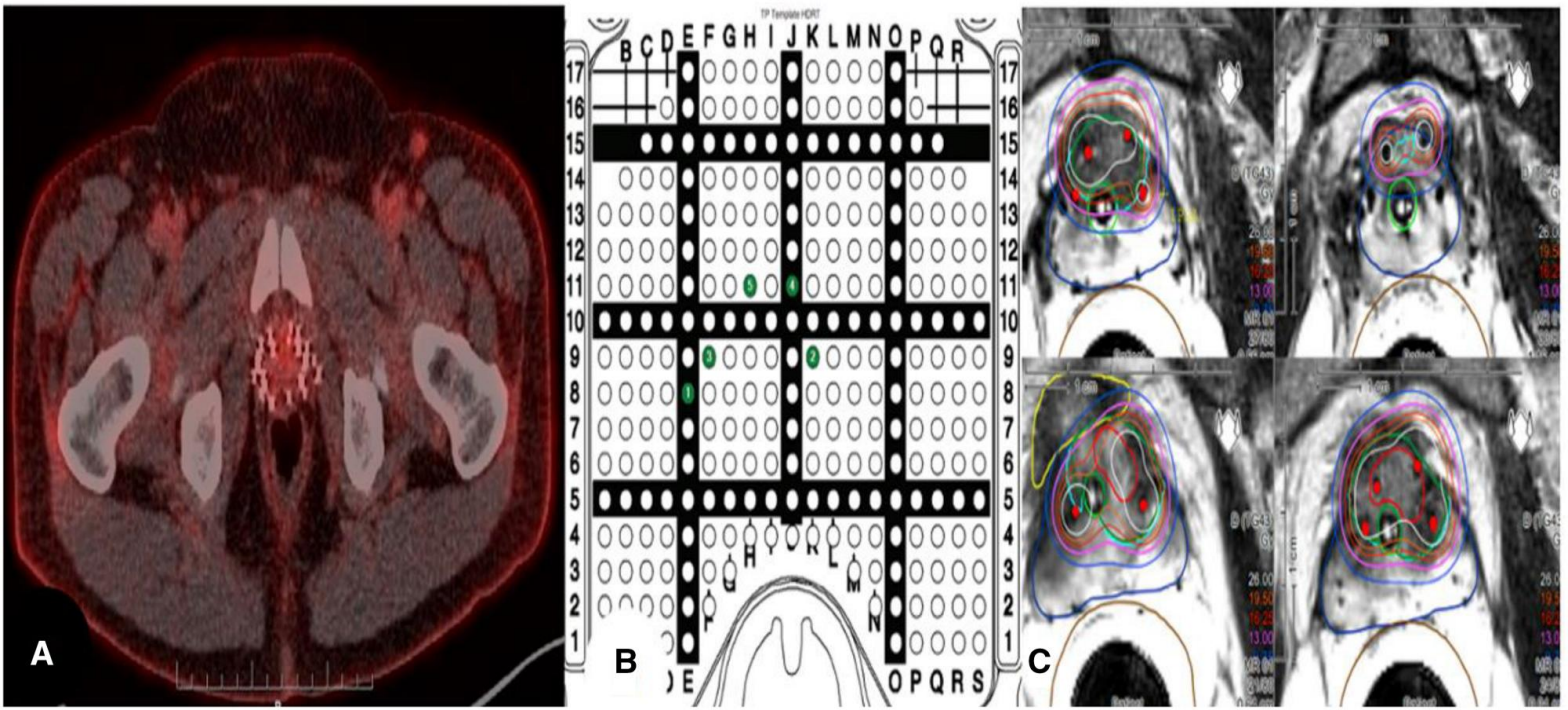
Salvage focal salvage HDR-BT in a patient previously treated with LDR-BT. (A) A prostate-specific membrane antigen positron emission tomography (PSMA PET) scan with an area of uptake at the anterior portion of the prostate apex; the biopsy of the lesion confirmed local recurrence. (B) The location of the catheter insertion by MRI guidance during focal salvage HDR-BT, and (C) Contoured in light blue PTV. Isodose lines: purple 13 Gy, orange 16.25 Gy, yellow 19.5 Gy.
